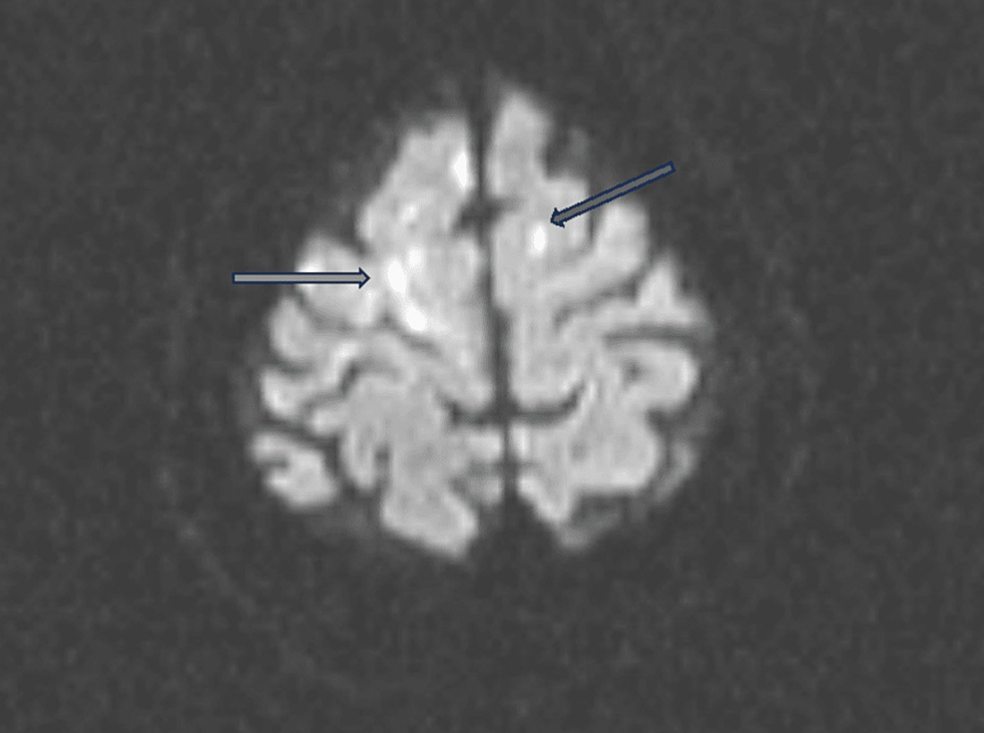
MRI showing multiple small-sized acute infarcts (arrow) MRI: magnetic resonance imaging
